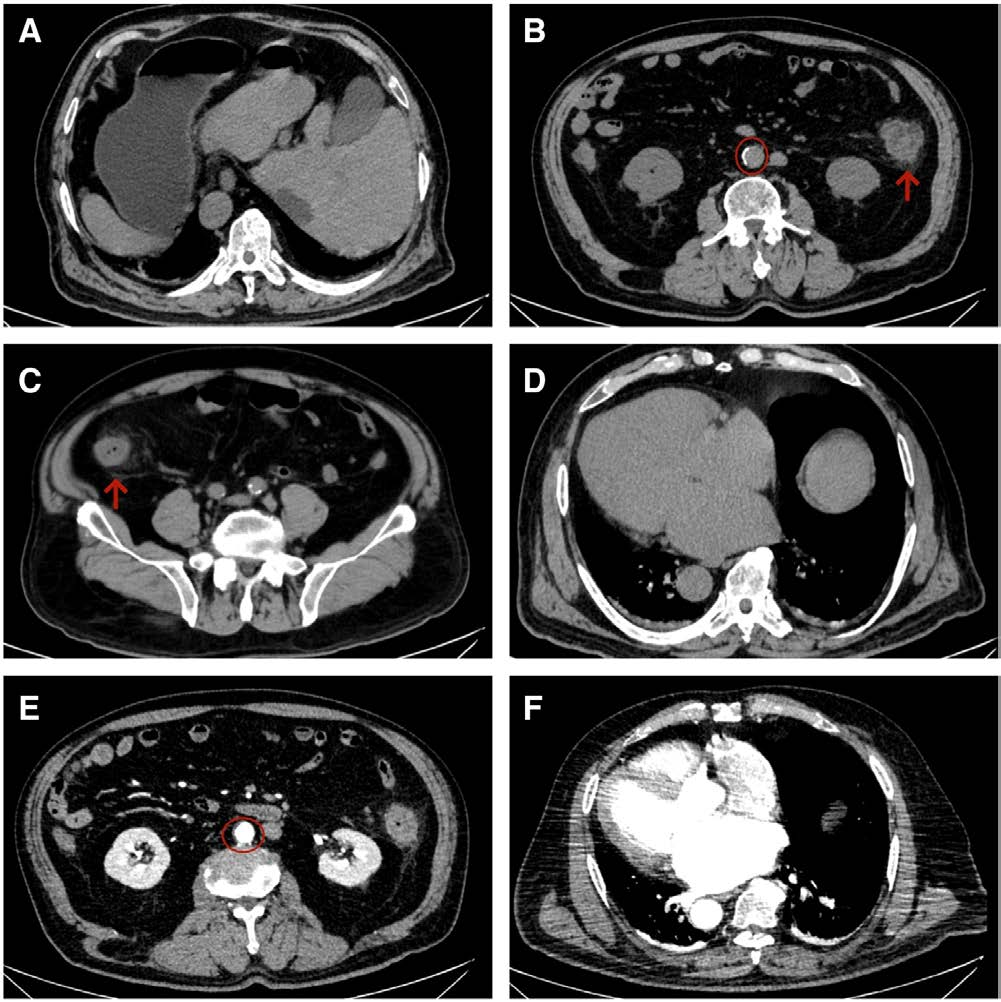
CT and CTA images. (A) CT scan results showed situs inversus totalis, localized thickening of the (B) left and (C) right colonic walls with blurred surrounding fatty spaces (red arrow), (D) and arc-shaped increased density shadows beneath the pleural membranes of both lower lung lobes. (E) Abdominal CTA revealed arteriosclerotic changes (red circle), (F) no abnormalities were detected in pulmonary CTA. CT = computed tomography, CTA = computed tomography angiography.
